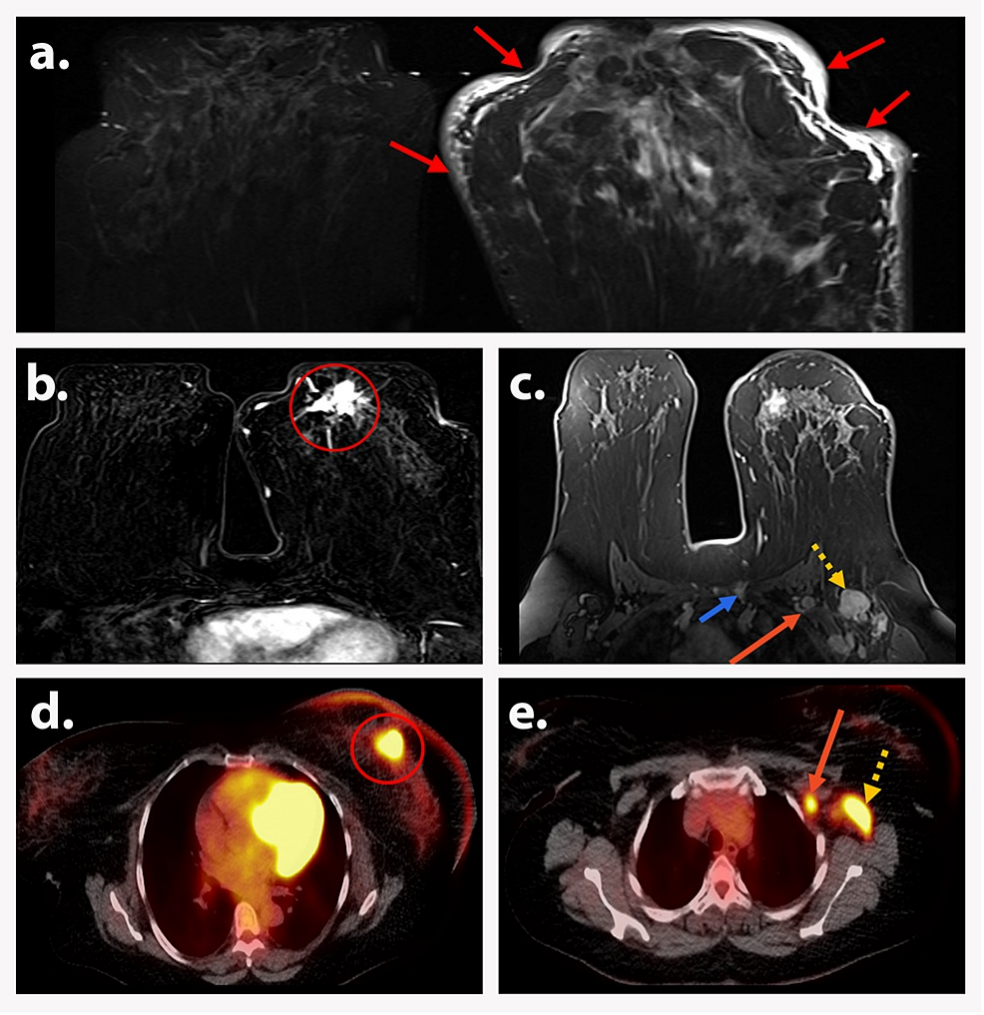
AJCC TNM Stage IIIC (T4dN3bM0) A 40-year-old premenopausal woman presented with left breast swelling and a palpable mass. Axial STIR image (a) shows left breast enlargement, diffuse edema, and skin thickening (red arrows). Axial T1-weighted subtraction DCE image (b) demonstrates a 1.8 cm irregular enhancing left retroareolar mass (red circle). Axial T1-weighted DCE image (c) shows level 1 (dotted yellow arrow) and level 2 (long orange arrow) left axillary lymphadenopathy and enlarged enhancing left internal mammary chain lymph node (short blue arrow). Axial PET/CT images (d and e) demonstrate FDG-avid skin thickening and edema and left retroareolar mass (red circle, image d), along with level 1 (dotted yellow arrow, image e) and level 2 (long orange arrow, image e). Biopsy depicted infiltrating ductal carcinoma. Findings are consistent with AJCC TNM Stage IIIC (T4dN3bM0). AJCC: American Joint Committee on Cancer; TNM: tumor, node, and metastasis; STIR: short tau inversion recovery; DCE: dynamic contrast-enhanced; PET/CT: positron emission tomography/computed tomography; FDG: fluorodeoxyglucose.
